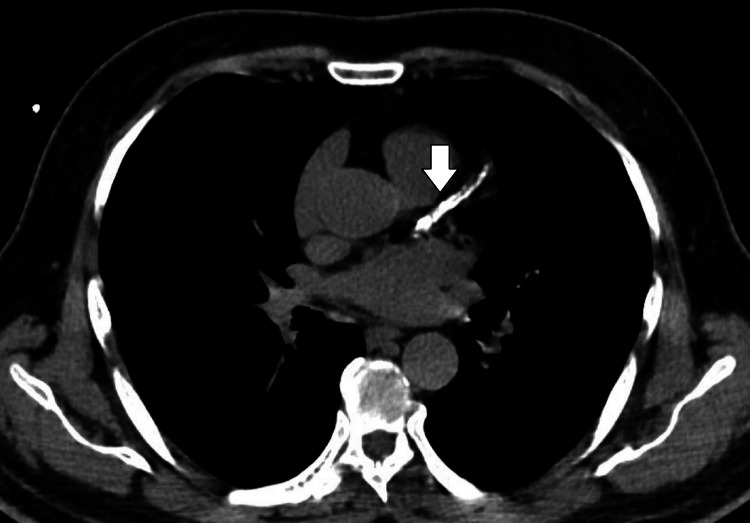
CT image of the LAD with a calcified lesion The white arrow represents the lesion. LAD, left anterior descending artery Image source: Alam et al. (2023) [10]
